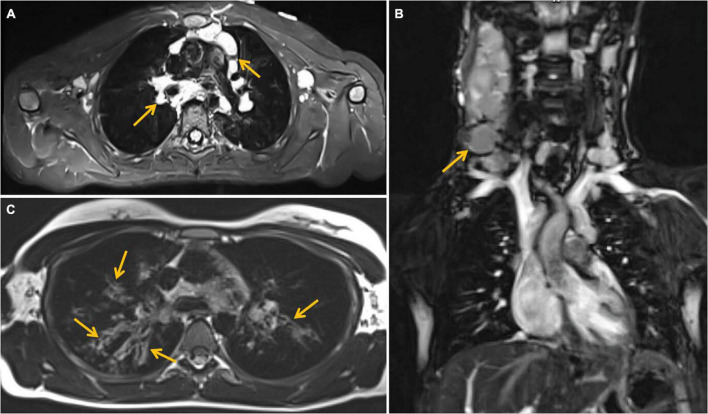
Magnetic resonance imaging (MRI) examination of the chest. (A) T2 -weighted Short-TI Inversion Recovery (STIR) axial image showing enlarged perivascular lymph nodes (marked with arrows), (B) coronal image—enlarged cervical lymph nodes (marked with an arrow). (C) T2-weighted blade axial image—bronchiectasis (marked with arrows) in upper lobes of both lungs, more severe on the right side.
